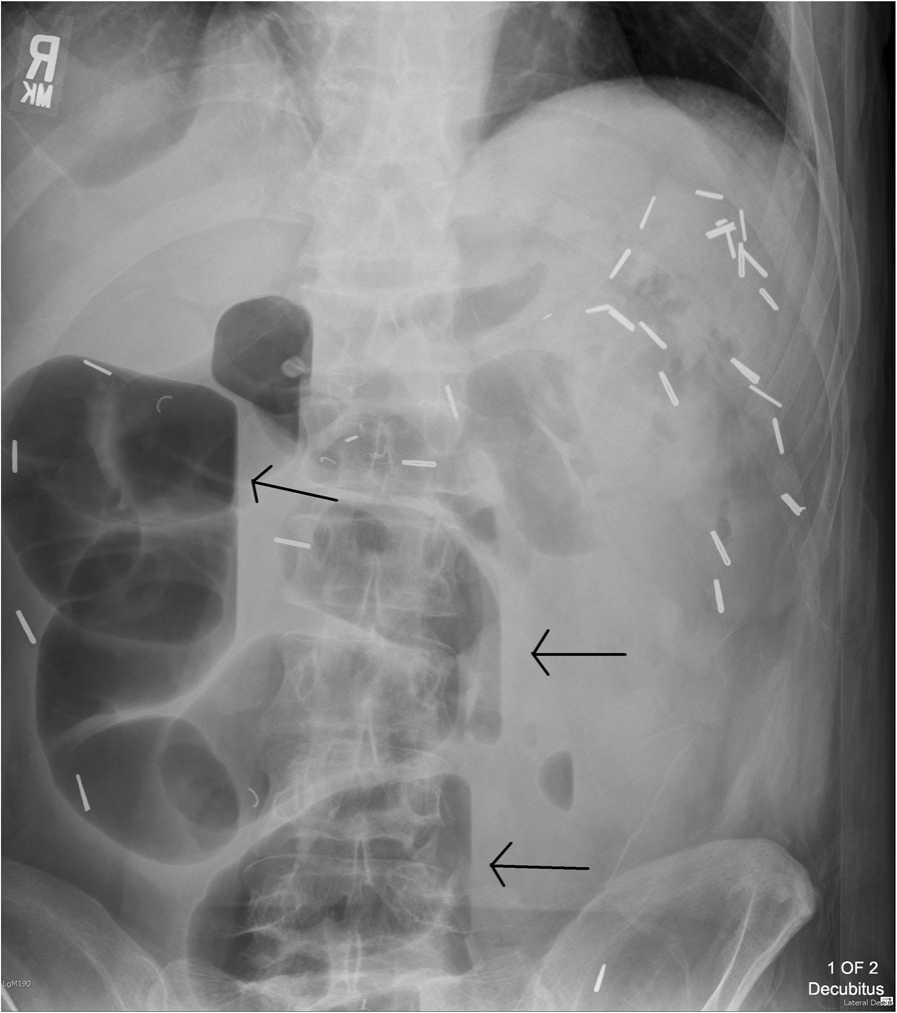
Left lateral decubitus radiograph demonstrating air-fluid levels. Incidental surgical clips from prior bowel resection are also noted.
